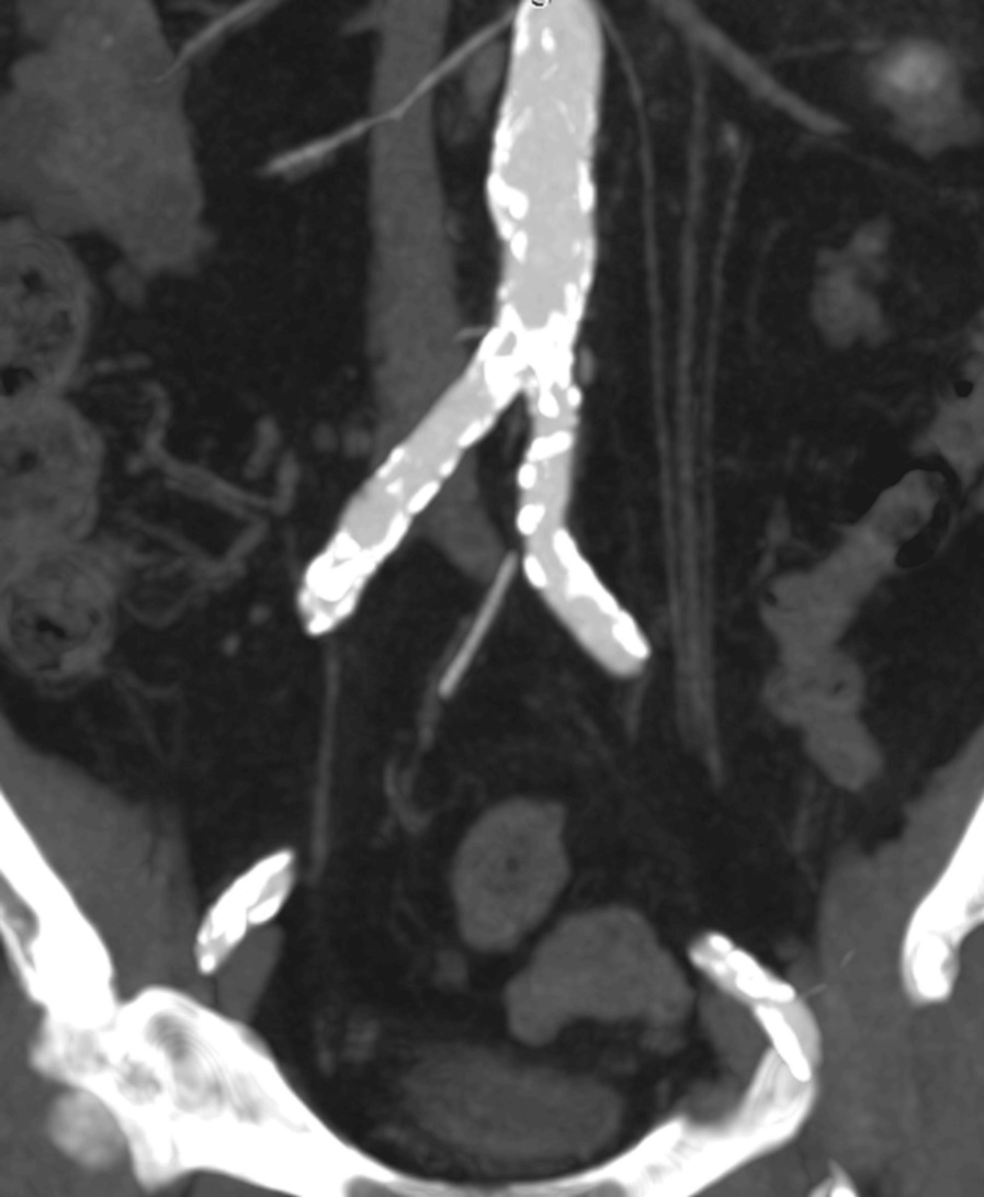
CT angiography demonstrating bilaterally heavily calcified CIAs. CT, computed tomography; CIAs, common iliac arteries
